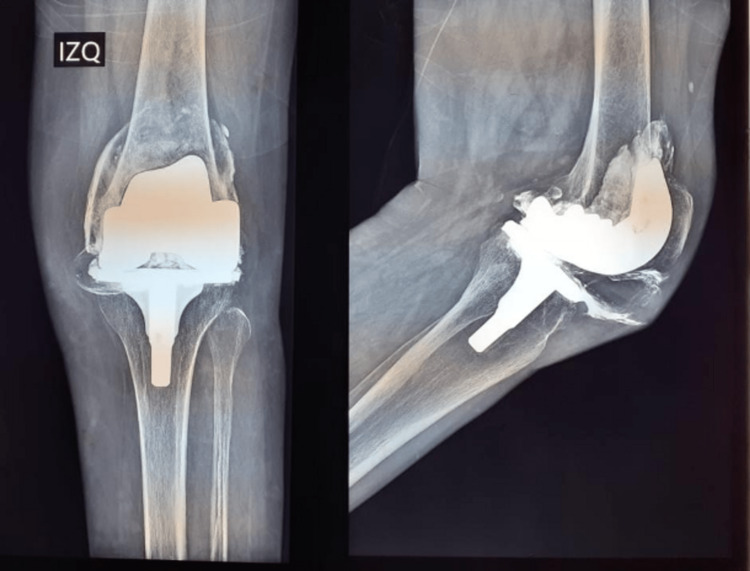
Radiograph images of the swollen knee Thin hyperdense line outlining a portion of the joint capsule which probably corresponded to the “metal line sign” observed metal-induced synovitis.
